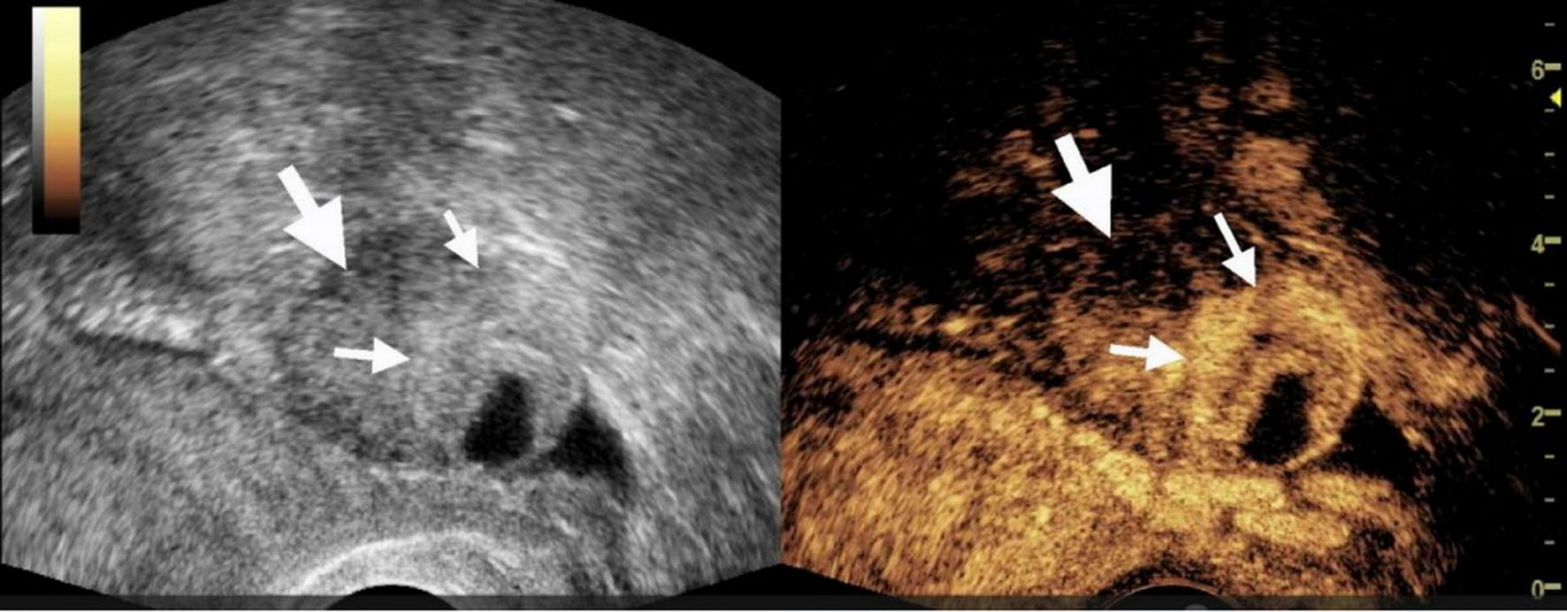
Transvaginal contrast enhanced ultrasound(CEUS) misdiagnosed the luteal structure in the ovary as an adnexal pregnancy. This was a case of intrauterine pregnancy misdiagnosed as ectopic pregnancy by CEUS. The patient, a 28-year-old woman, visited the hospital due to abdominal pain. Her blood HCG level was 84 mIU/mL. The left image showed a high-echo mass (thin arrow) near the left ovary (thick arrow), measuring 2.2cm×2.0cm. The right image of CEUS showed significant enhancement of the mass with a crescent moon shape. The enhancement of the ovary was less than the mass. The ultrasound diagnosis was ectopic pregnancy with the location to be undetermined and the possibility of ovarian pregnancy was relatively high. The patient was later confirmed to have anuterine pregnancy. After a curettage, the patients HCG levels returned to normal and no abnormalities were seen in the ovaries
